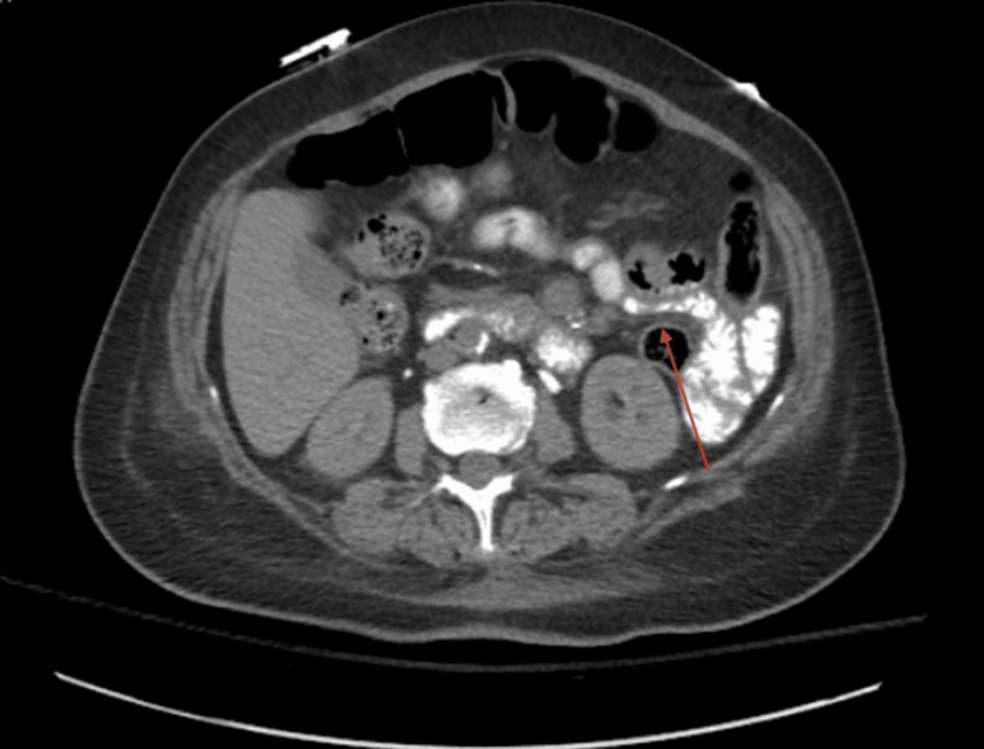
A multi-detector CT imaging of the abdomen and pelvis was performed using the standard protocol bolus administration of oral contrast, which showed abnormal dilated loops of small bowel wall thickening (red arrow).
